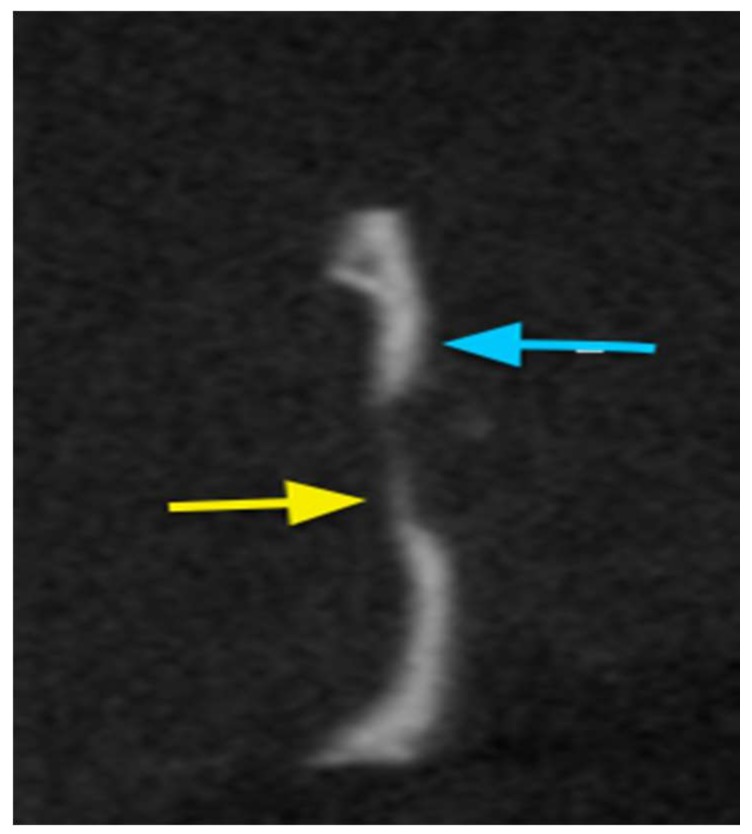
Sagittal view for cone beam computed tomography (CBCT) for the coated PE disc in situ after three months. The area of bone regeneration is shown by the yellow arrow, while the area of native bone is shown by the blue arrow. The area of the PE graft appears radiolucent.
